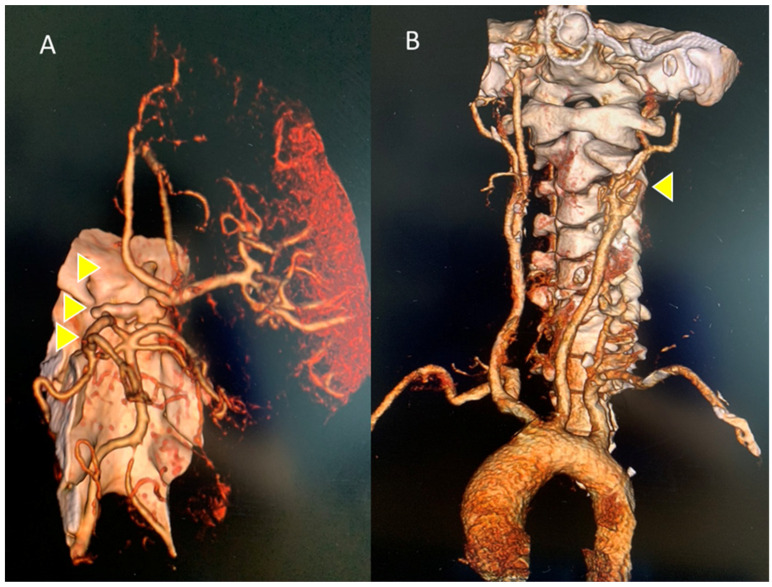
Intracranial and epiaortic angio-CT: (A) left anterior circulation occlusion: intracranial internal carotid, middle cerebral (MCA), anterior cerebral arteries; (B) lack of opacification on the left of the internal carotid artery a few mm from the origin. Thrombotic occlusion areas are indicated by yellow arrows.
